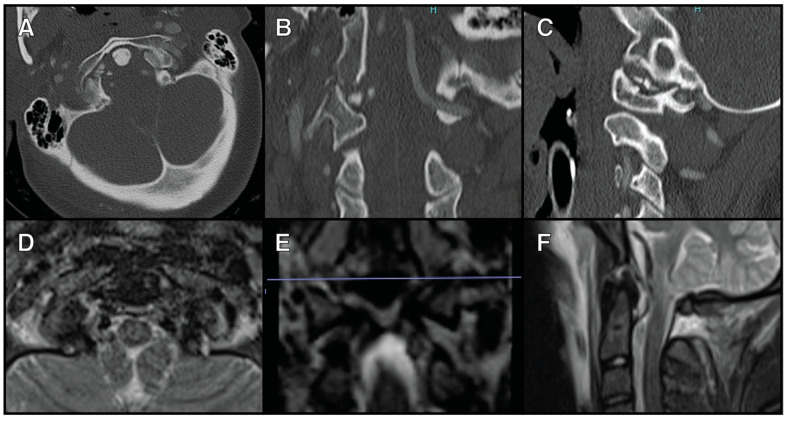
A 27-year-old female who presented after a motor vehicle collision with a right type III OCF and C1 lateral mass fracture (A) The axial view of a CTA scan showing the right type III OFC. (B) The coronal view of a CTA scan showing the right type III OFC. (C) The sagittal view of a CTA scan showing the right type II OFC. (D) The axial view of an MRI T2 STIR sequence showing right alar ligament disruption. (E) The coronal view of an MRI T2 STIR sequence showing right alar ligament disruption. (F) The sagittal view of an MRI T2 STIR sequence showing posterior ligamentous disruption.
